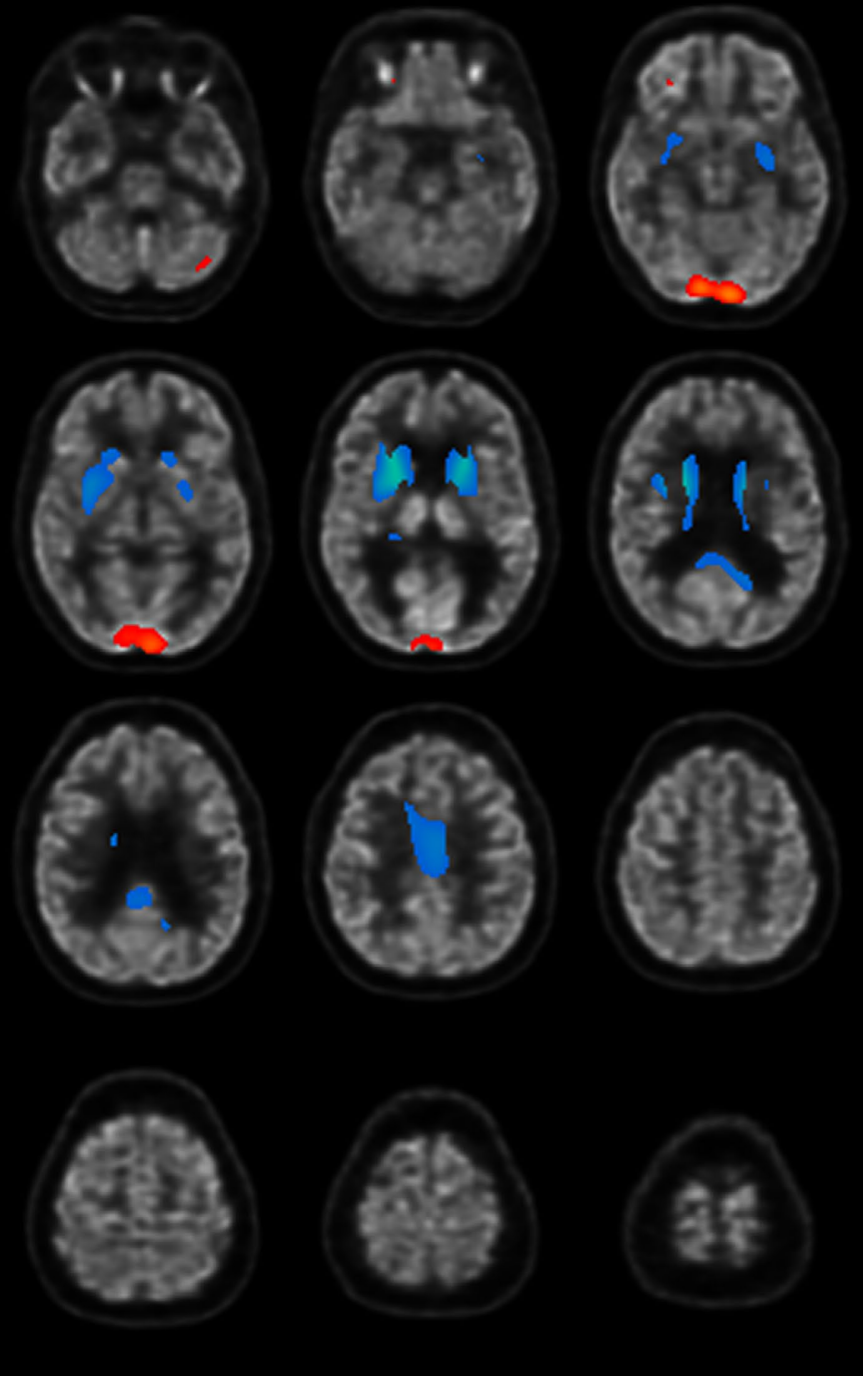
Statistical parametric maps overlaid to 18F‐FDG PET/CT images showing striatal hypometabolism and occipital hypermetabolism
